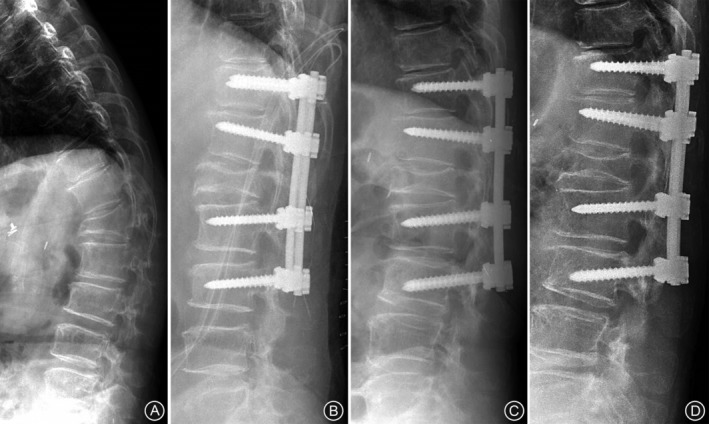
(A) Preoperative radiograph of a 65‐year‐old woman with an L_1_ osteoporotic compression fracture showing a kyphotic angle of 52.1 degrees. (B) Immediate postoperative radiograph showing kyphosis correction to 6.3 degrees. (C) The kyphosis progressed to 18.6 degrees at 2 years postoperative. (D) Lateral plain radiograph taken at 4 years postoperative showing well maintained sagittal correction with kyophosis of 19.7 degrees.
